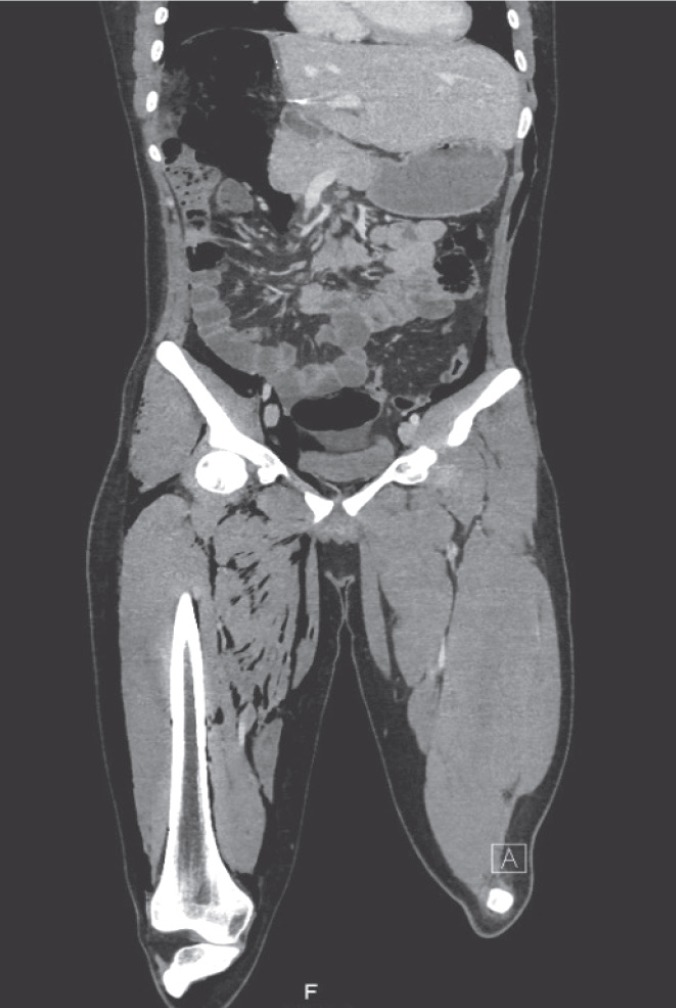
The coronal CT cross-section demonstrates massive subcutaneous emphysema, pneumoperitoneum and pneumoretroperitoneum
